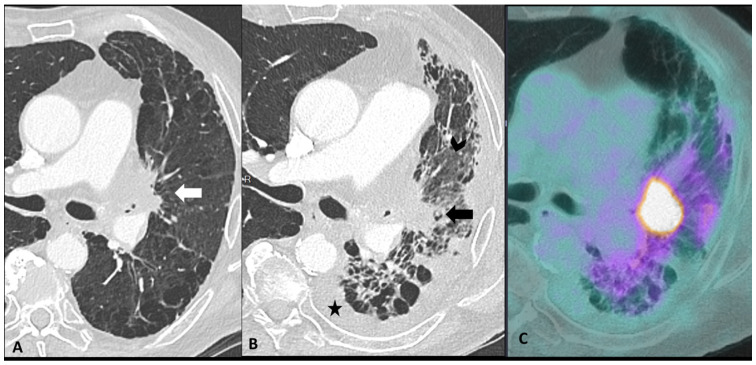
Mimicker of immune-related pneumonitis: tumor progression in a patient with unresectable stage III receiving consolidation immunotherapy after chemoradiotherapy. At baseline CT (A) the peri-hilar lesion appearance after radiation therapy is seen (arrow). The restaging CT four months after the introduction of antiPD-1 (B) shows the appearance of an extended ground-glass opacity (arrowhead) and septal thickening in the left lower lobe as well as the increase in the size of the peri-hilar mass (arrow) and a semi-circumferential pleural effusion (star), findings suspicious for tumor progression with lymphangitic involvement rather that immune-related or radiation-related pneumonia. The intense uptake of the peri-hilar lesions at PET/CT (C) confirms the tumor progression.
